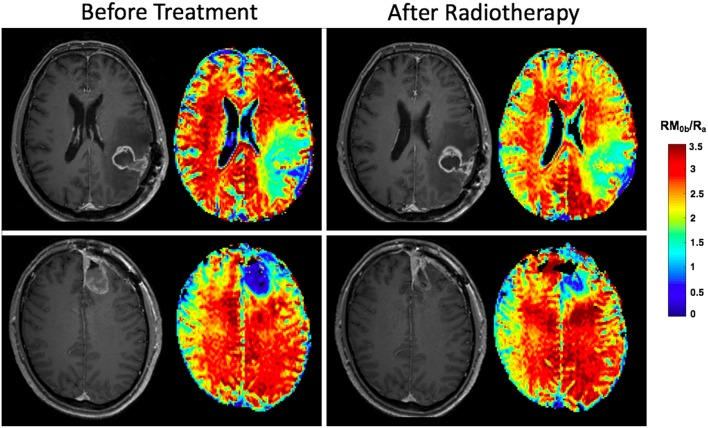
Effects of radiotherapy plus Temozolomide on normal tissue of two patients with GBM. Both patients received IMRT at a dose of 2Gy/day. After the first 10 fractions, the white matter on the contralateral side of the brain on post-Gd T_1w_-MRI appears normal before and after treatment for both patients. The parametric maps show the amount of magnetization transfer (MT), RM_0b_/R_a_ which quantifies white matter integrity. The patient in the top row experiences significant decrease in amount of MT showing significant white matter damage, while for the patient in the bottom row, the amount of MT has not changed showing the patient's resistance to radiation.
